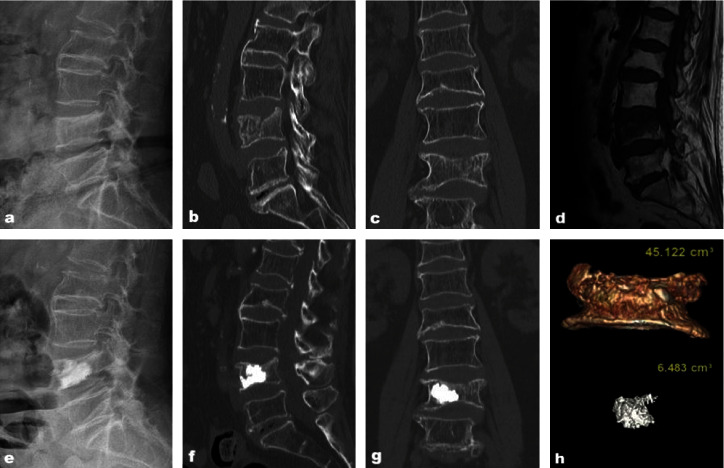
An 80-year-old female patient is diagnosed with an L4 osteoporotic vertebral compression fracture (OVCF). (a) Preoperative lateral X-ray. (b) Preoperative sagittal CT. (c) Preoperative coronal CT. (d) Preoperative MRI. (e) Postoperative lateral X-ray. (f) Postoperative sagittal CT. (g) Postoperative coronal CT. (h) 3D image of the vertebral body and bone cement.
